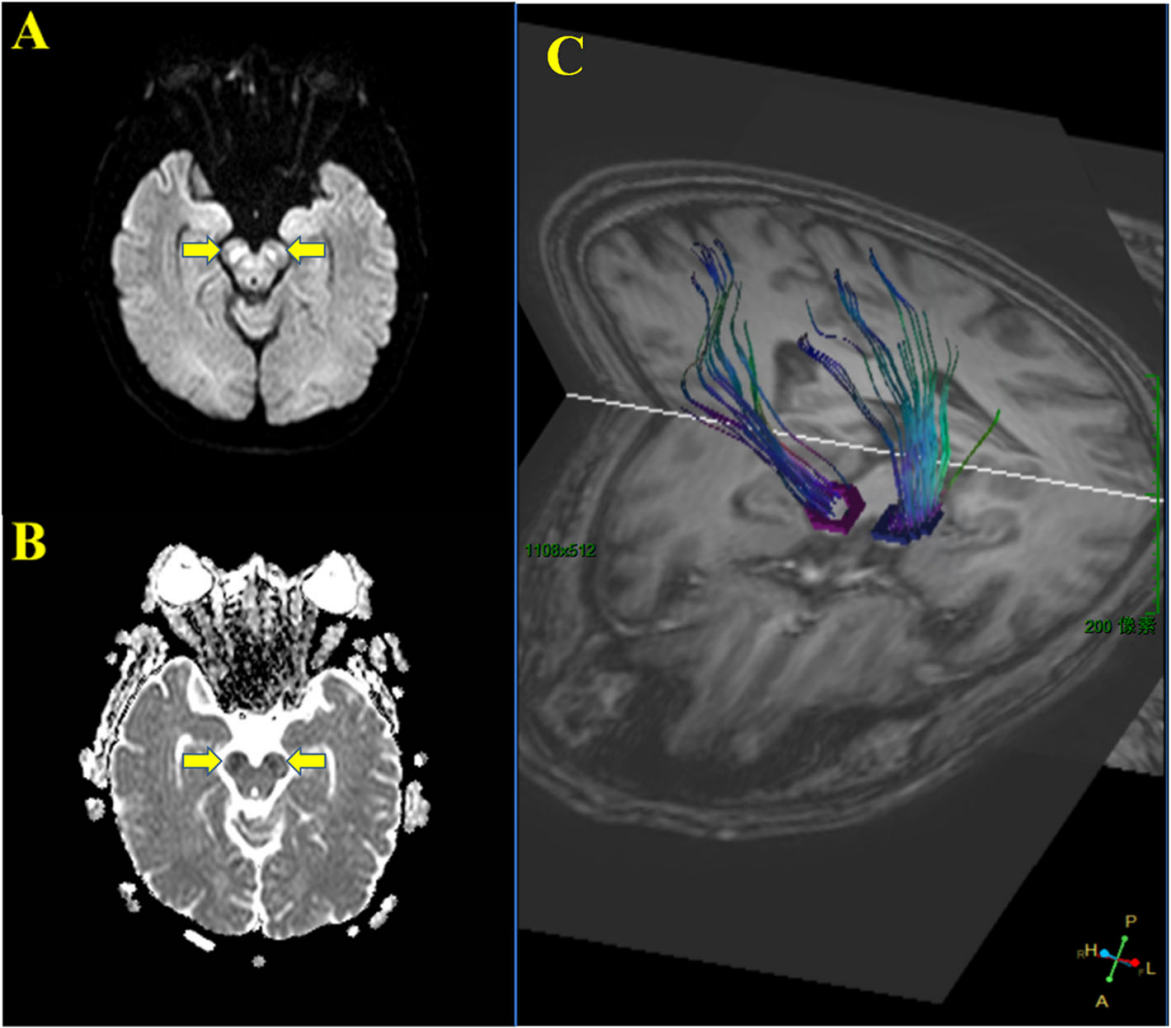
Similar to case 1, bilateral cerebral peduncles showed symmetrical high signals in DWI (a), but low signals in ADC (b). Wallerian degeneration of bilateral cerebral peduncles secondary to corticospinal tract injury was confirmed by DTI.
